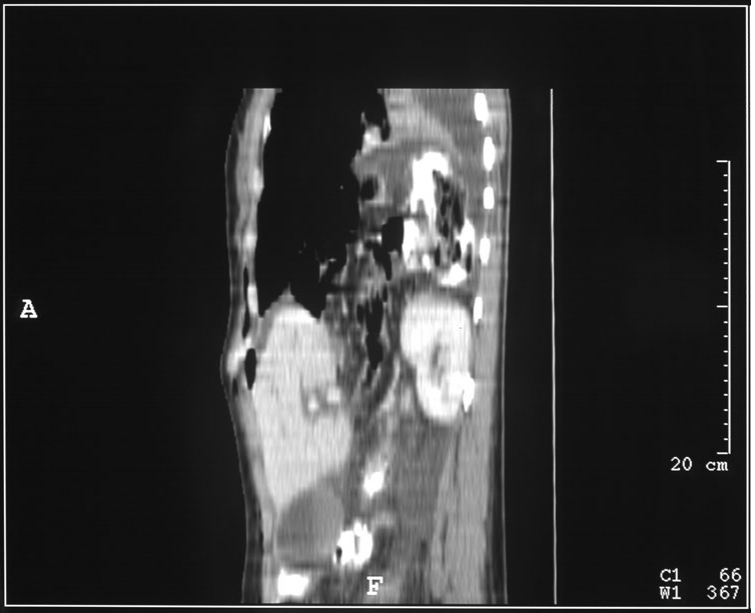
Three-dimensional CT reconstruction demonstrating the diaphragmatic defect
